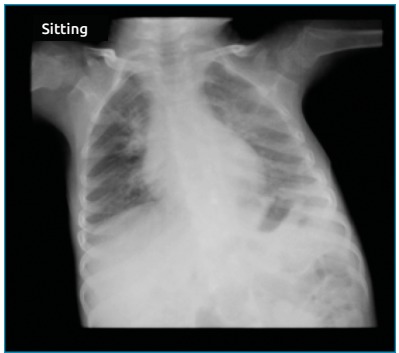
Chest X-ray performed during the follow-up with no
